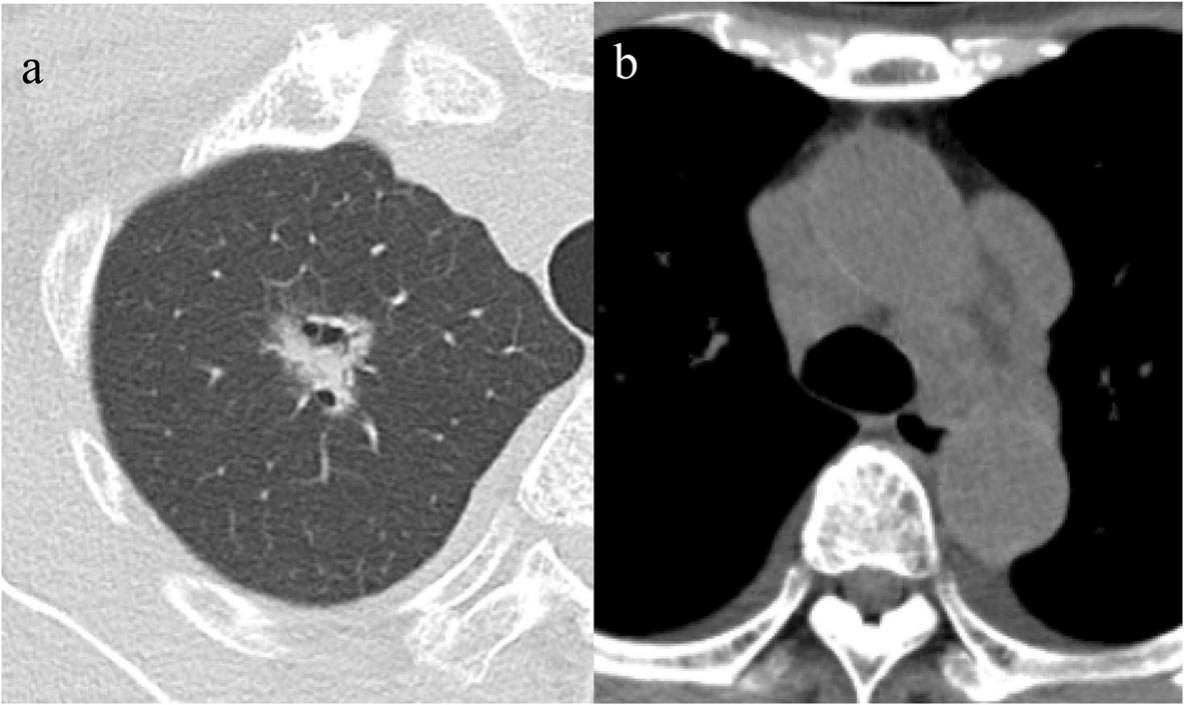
Computed tomography (CT) images. a Chest CT scan showing the nodule with marginal irregularity and bronchodilatation in the right upper lobe. b CT scan in the mediastinal window showing mediastinal lymph node enlargement and bilateral pleural effusion
